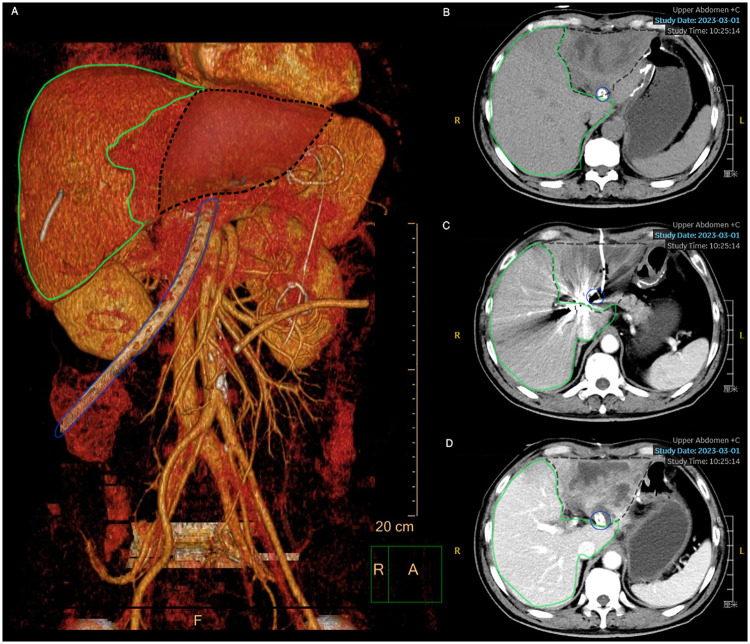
Three-dimensional contrast-enhanced CT series of liver abscess following liver ischemia post-emergent DSA in 2 weeks. (A) Volume rendering of liver and primary operation area; blue line indicates double-space irrigation tube; (B–D) contrast-enhanced CT of liver abscess in plain scan/arterial phase/portal venous phase; green line indicates normal area of liver; black dash indicates liver abscess resulting from liver ischemia post DSA with common hepatic artery and proper hepatic artery embolized.
